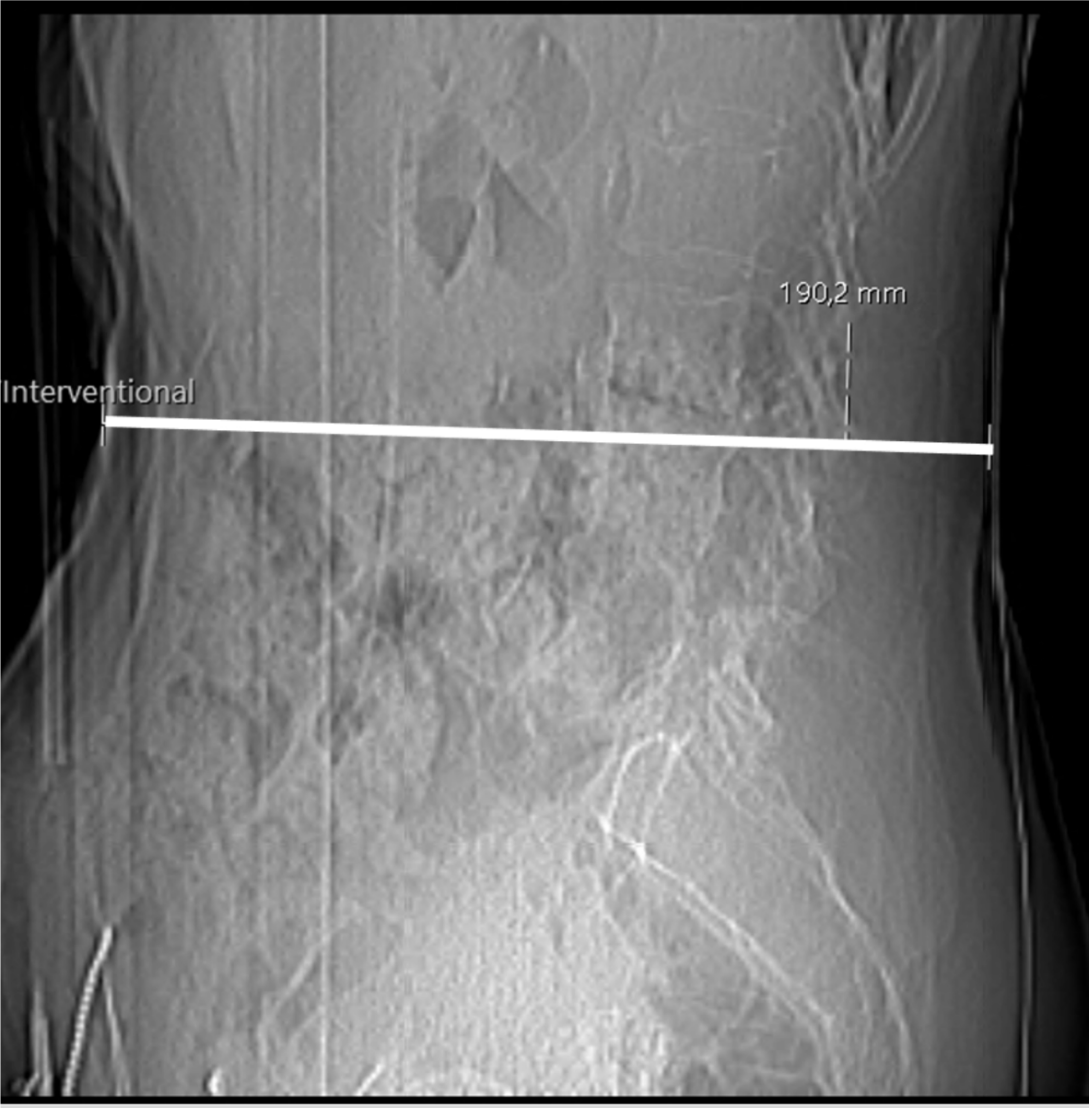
Lateral scout obtained for a planned computed tomography (CT) myelography in an 83-year-old female patient. The line indicates the anterior-posterior body diameter, which was equal to 190.2 mm in this exemplary case.
